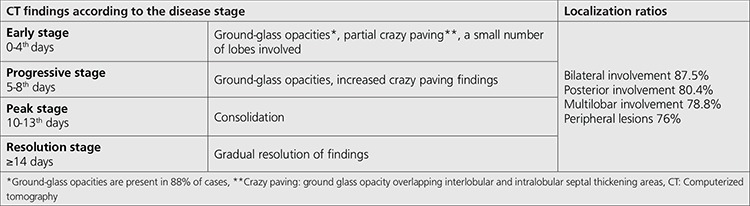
CT findings and their distributions in the lung lobes that can be observed during COVID-19 infection
